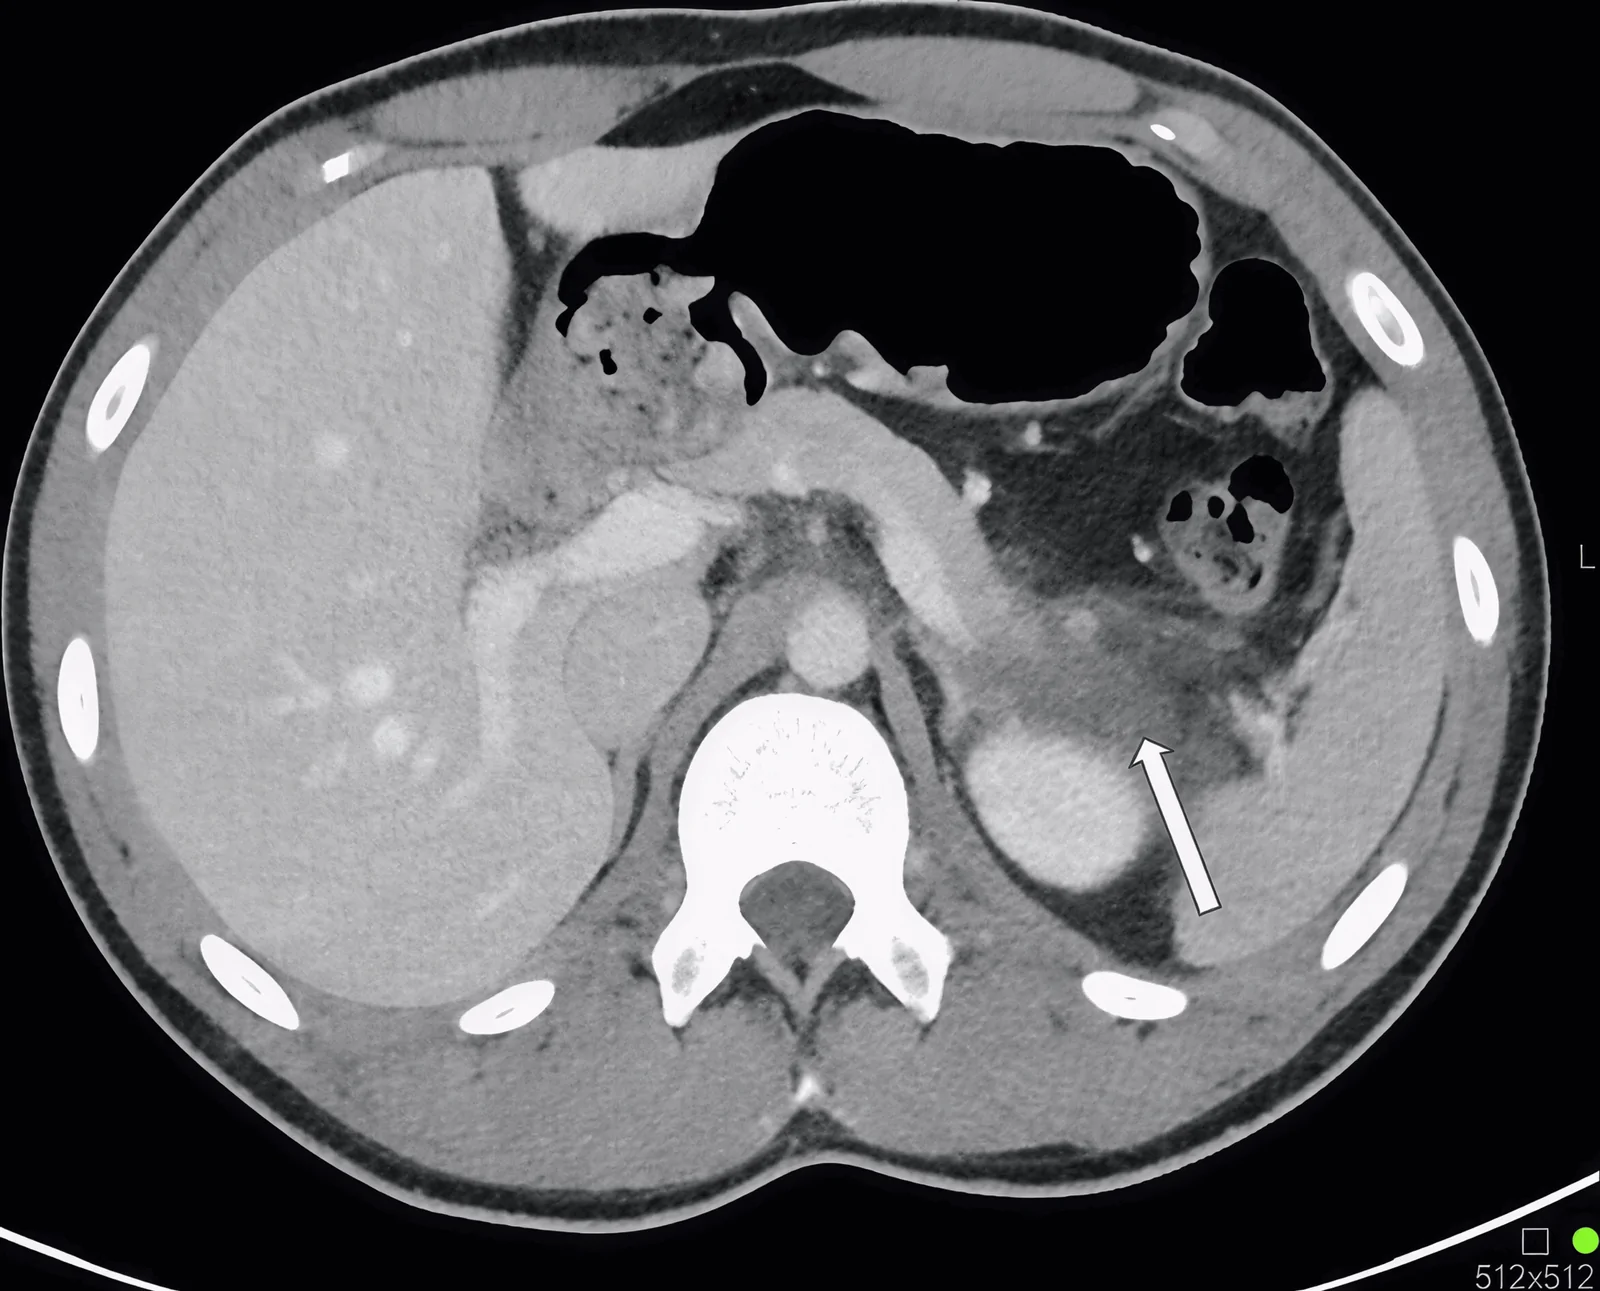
CT scan demonstrating localized inflammatory stranding and trace fluid surrounding the pancreatic tail. The arrow points to the area of inflammatory stranding and trace fluid around the pancreatic tail.
